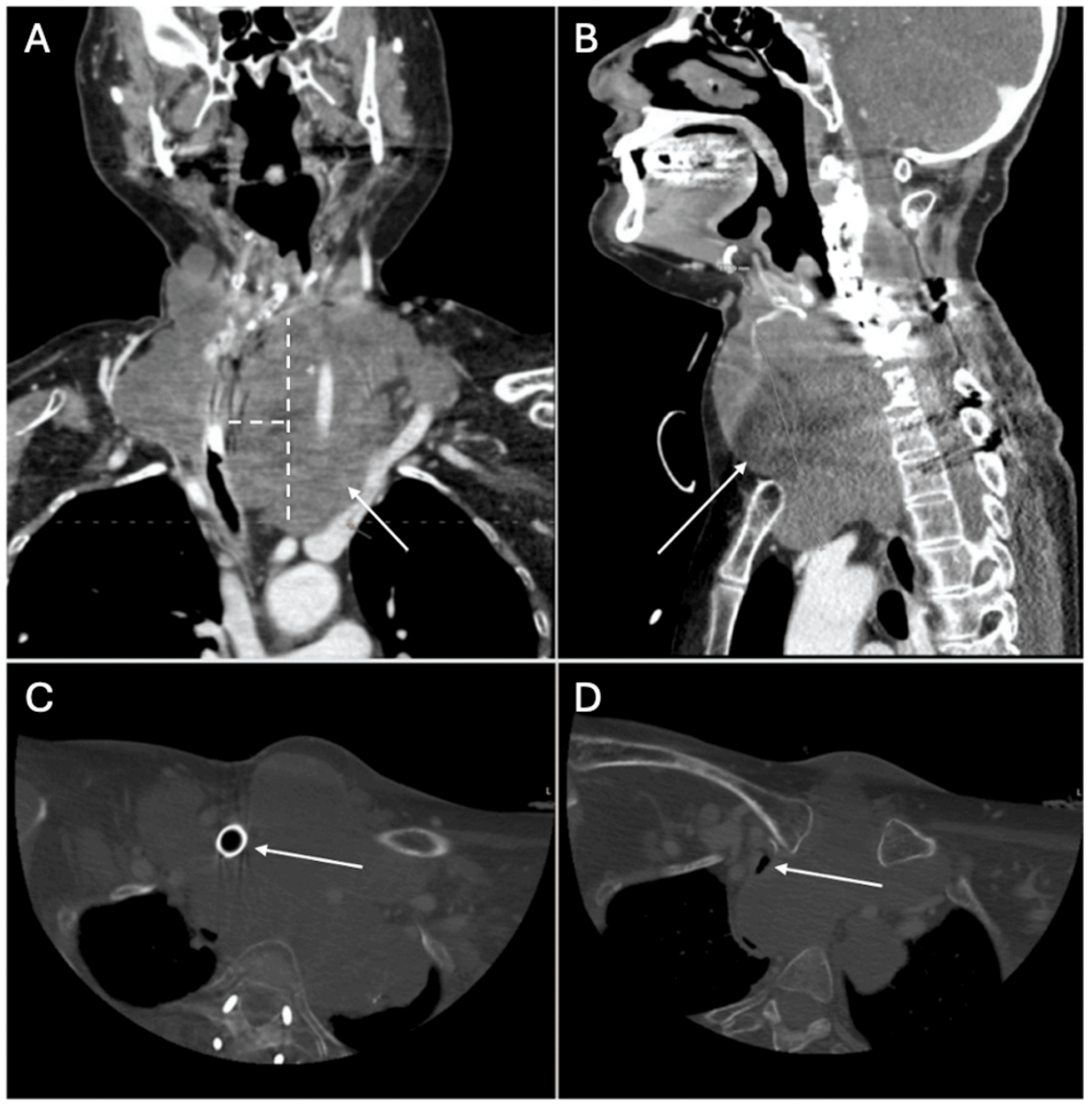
Head and neck CTA Sagittal (A), Coronal (B): High attenuation fluid is noted in the pharynx, suggesting the presence of blood. Similar appearance of the transspatial chordoma throughout the cervical spine and neck compared to prior imaging with chronic bilateral carotid and vertebral artery encasement, severe rightward deviation of the airway, and effacement of the esophagus. The tracheostomy tube courses through the mass with persistent narrowing of the airway distal to the tracheostomy tube. CT axial views (C, D): Tracheostomy tube visualized (C). Airway patency at the next slice distal to the tracheostomy tube diameter of 7 mm (D). CTA: computed tomography angiography
